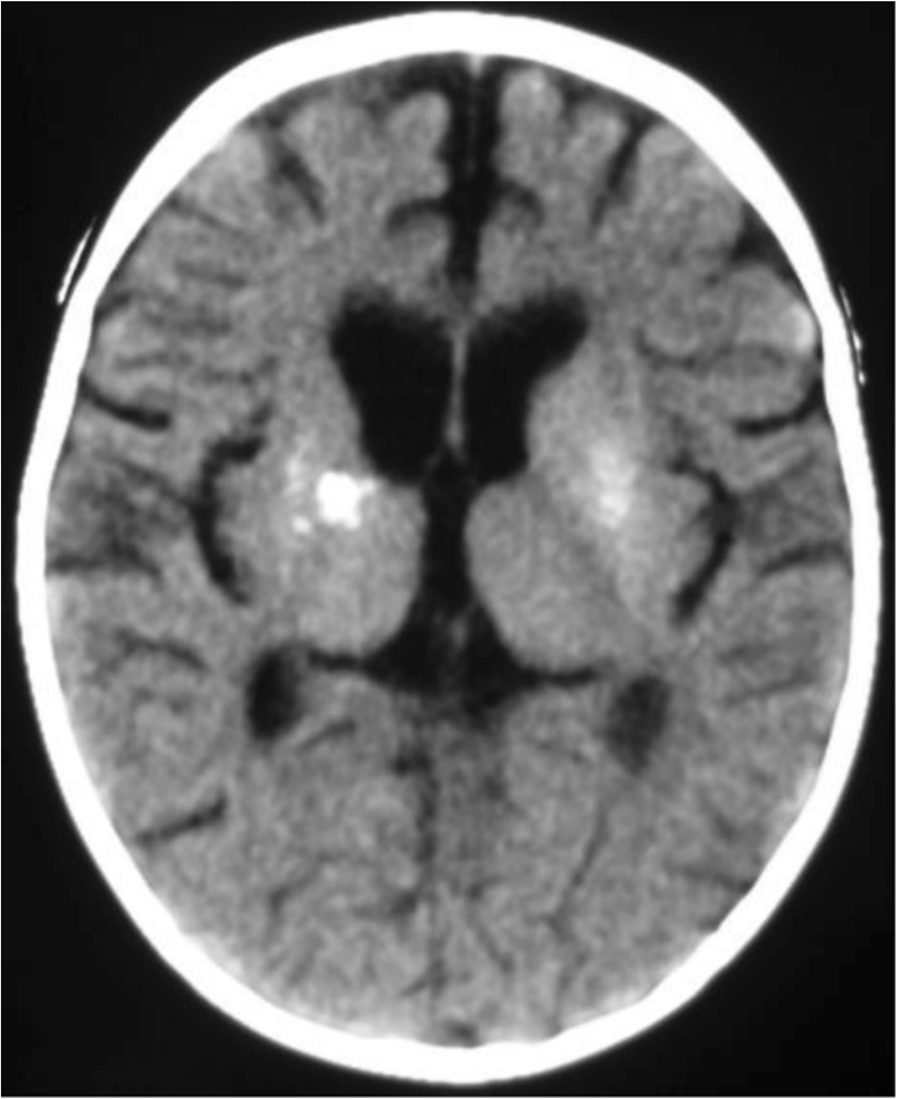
Axial CT Brain showing bilateral basal ganglia calcification and cerebral shrinkage in a 1year old child with HIVE.
